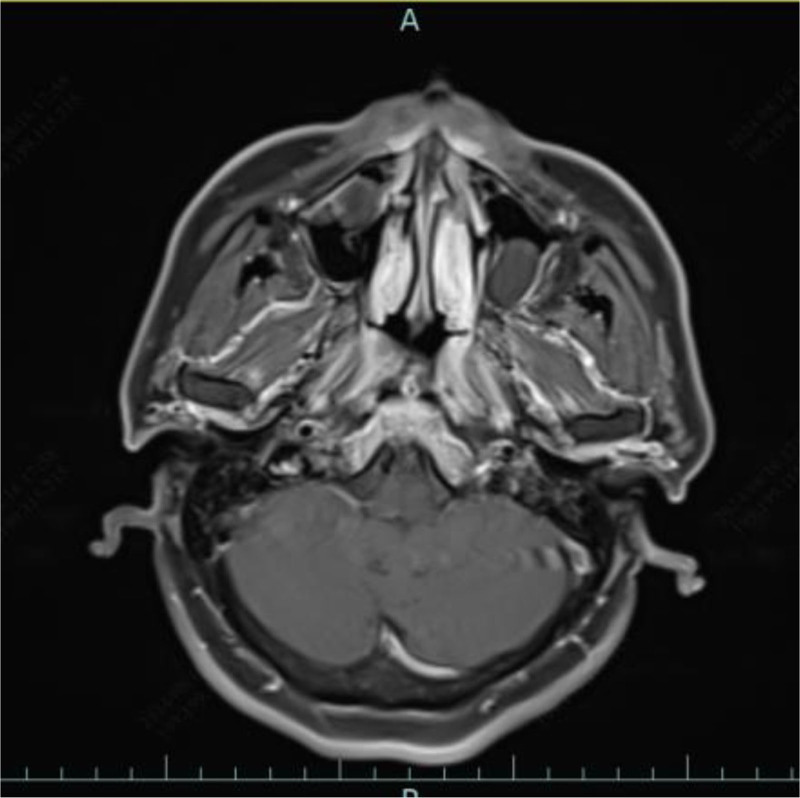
Reexamination of nasopharyngeal enhanced MRI suggested that the nasopharyngeal mucosa was slightly thickened and there was no obvious enhancement. MRI = magnetic resonance imaging.
